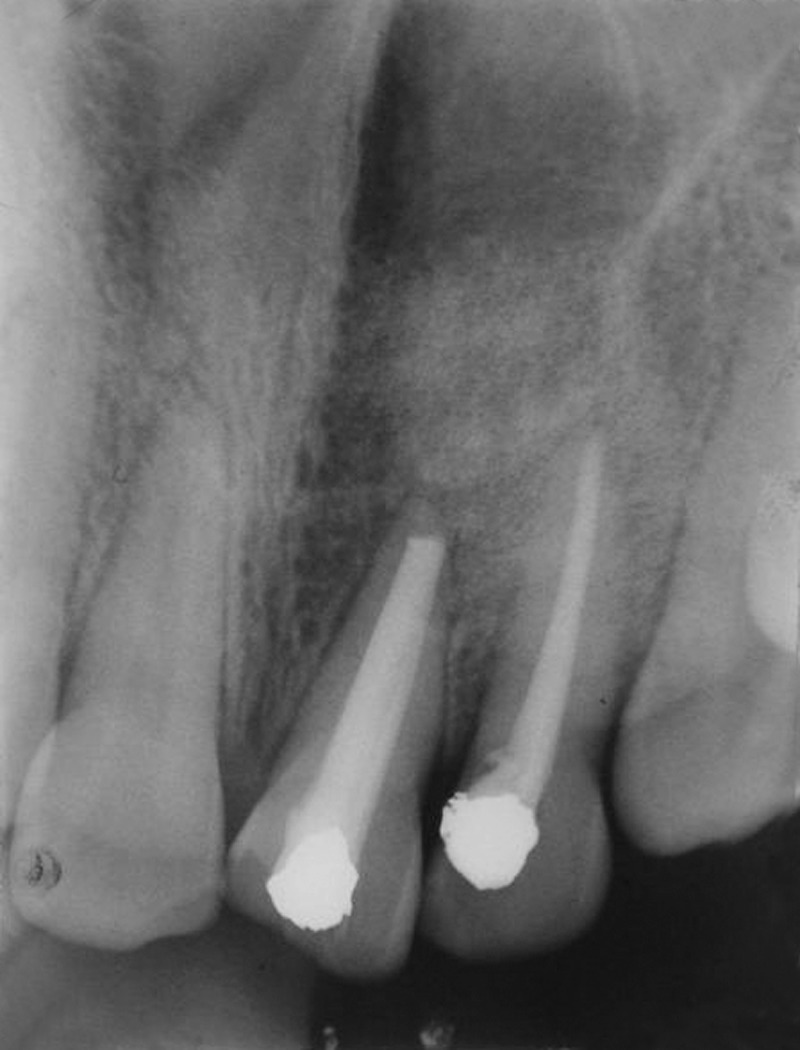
Six months postoperative X-ray
